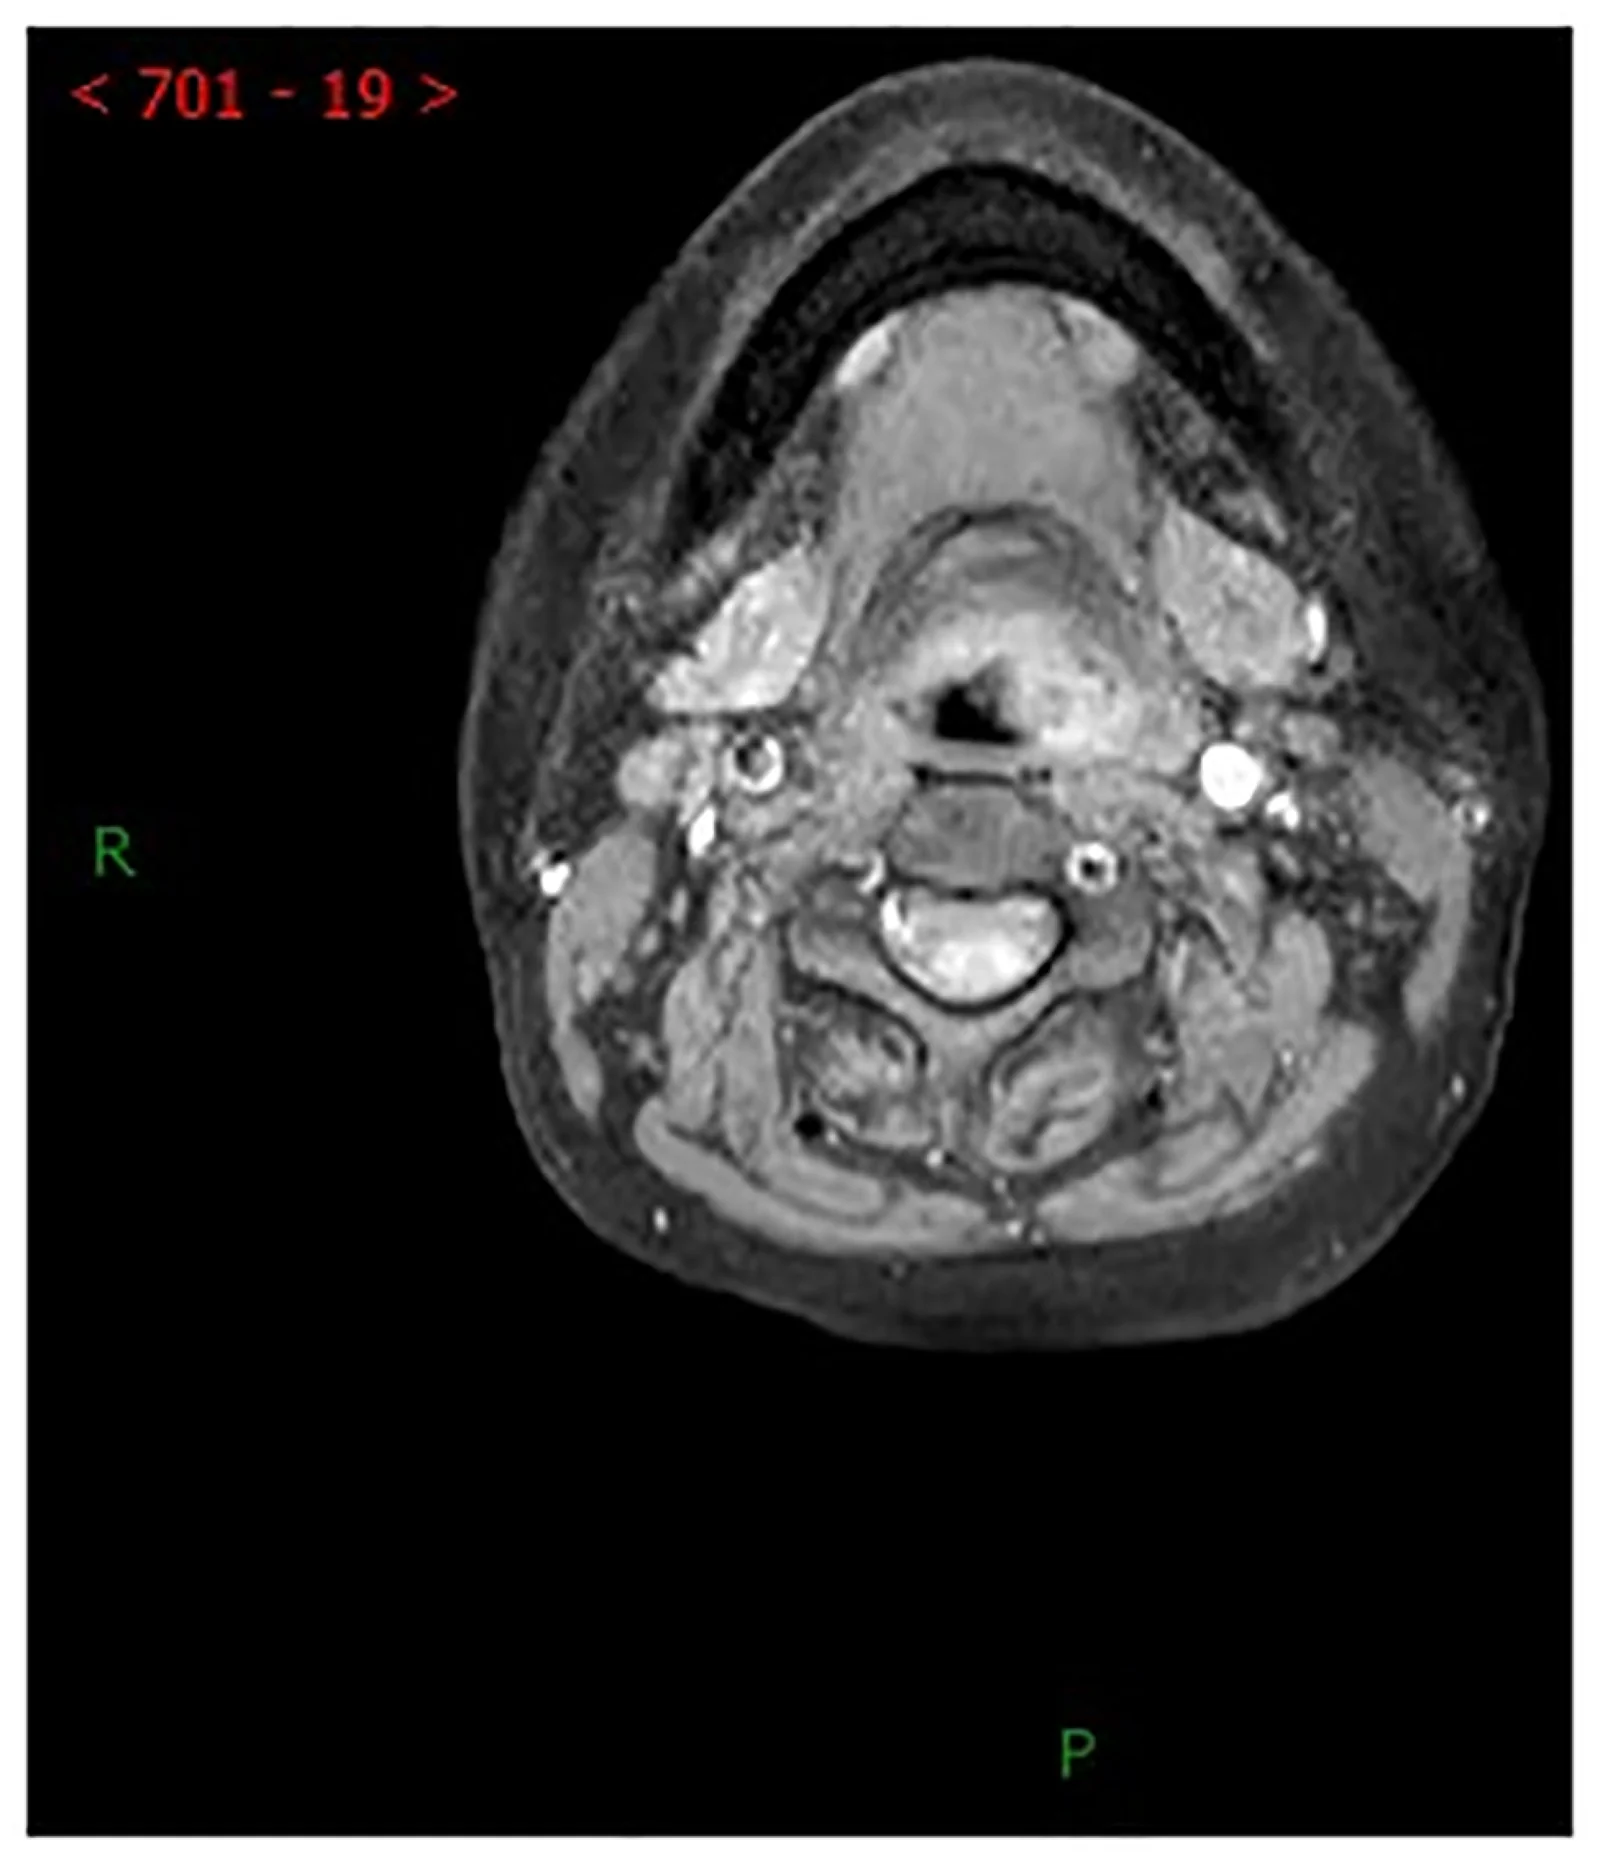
Neck MRI findings. A mass in the left pyriform sinus is shown. MRI = magnetic resonance imaging.
